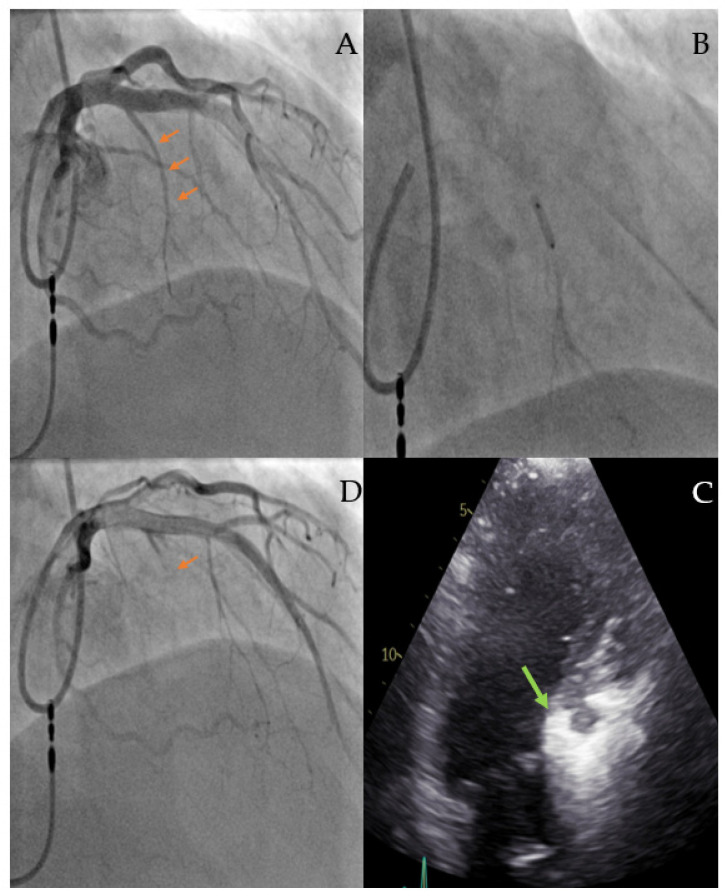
Procedural steps of alcohol septal ablation. (A) Angiogram of the left coronary artery (red arrow indicates the first septal branch as the target for ASA); (B) Selective angiogram of the first septal branch, which is occluded with an over-the-wire balloon; (C) Myocardial contrast echocardiogram verifying the target region (arrows indicate the highlighted septal region); (D) Final angiogram of the left anterior descending coronary artery after alcohol injection (arrow indicates the occluded septal branch).
